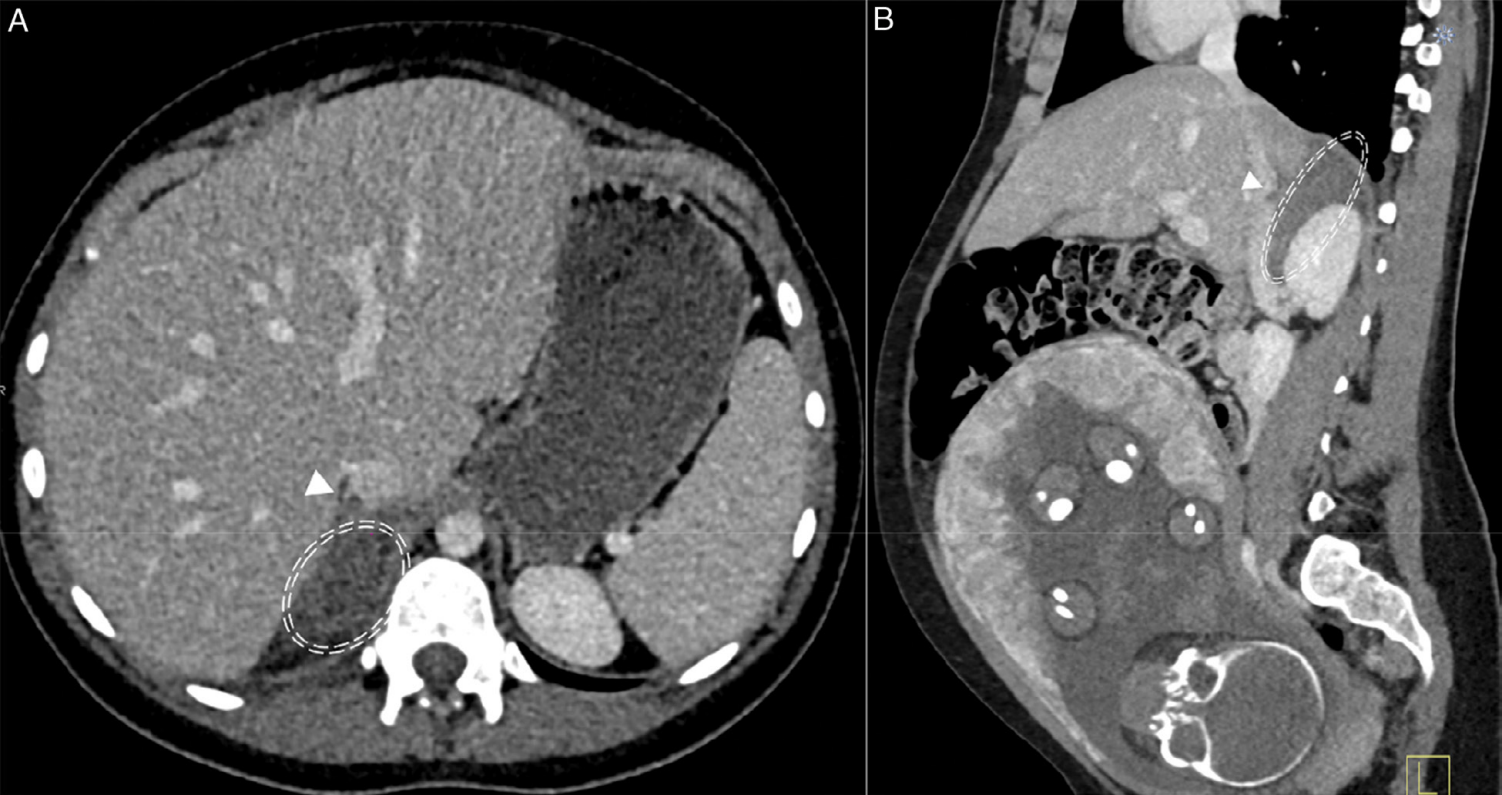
Axial (A) and sagittal (B) plane abdominal CT at portal phase showing poor enhancement and swollen appearance of the right adrenal gland (circle) and hypodense filling defect within the inferior vena cava (arrowhead), corresponding to the adrenal vein thrombosis overflowing in the inferior vena cava.
